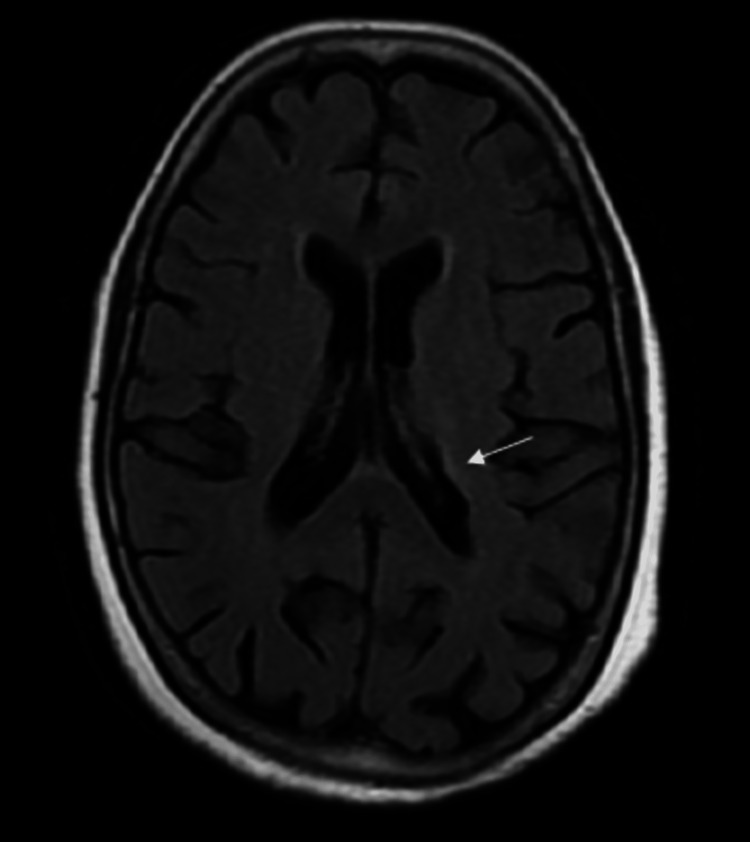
T2 FLAIR image of brain MRI showing hyperintense signal in the left corona radiata (white arrow). MRI, magnetic resonance imaging; FLAIR, fluid-attenuated inversion recovery
